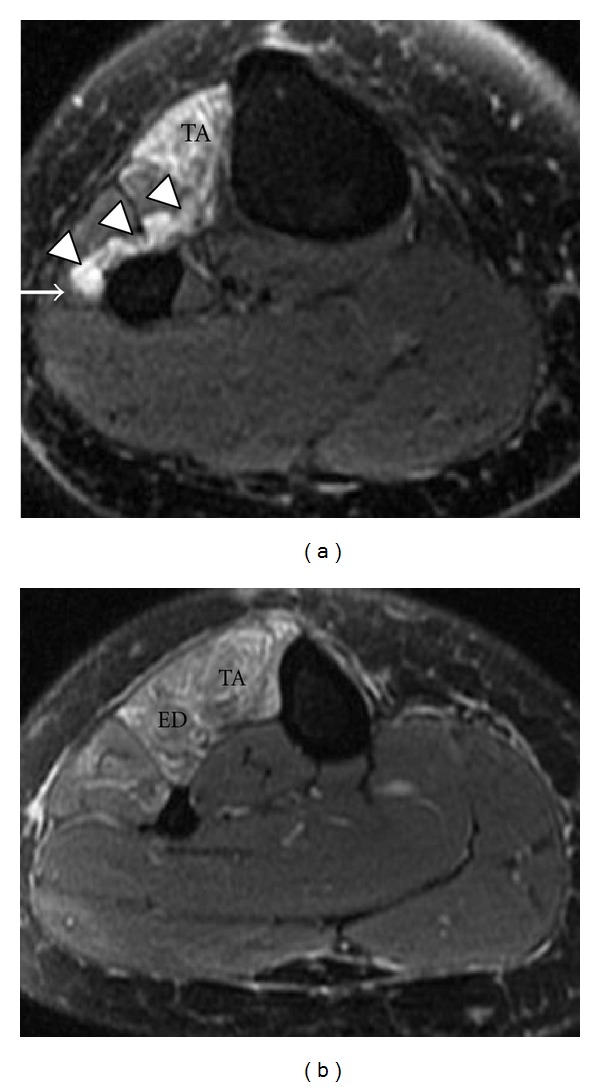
Common peroneal nerve entrapment secondary to a surgically proven intraneural ganglion cyst in a 44-year-old patient with a 6-month history of right foot drop. Axial T2-weighted fat-saturated images (a, b) reveal a multilobulated high T2 signal structure (arrowheads) compressing the adjacent common peroneal nerve (arrow). Associated patchy high signal in tibialis anterior (TA) and extensor digitorum longus (ED) muscles.
